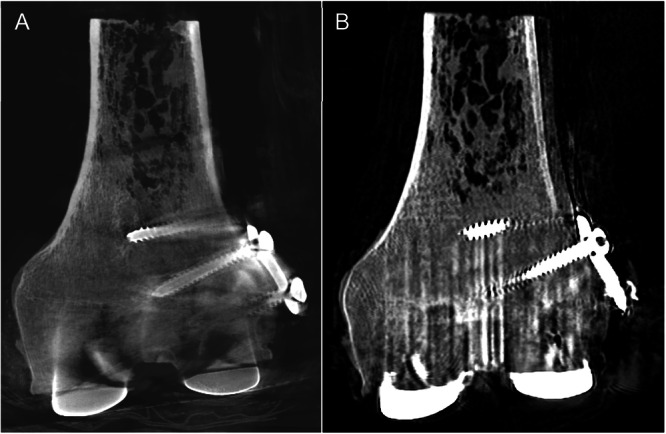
CT scans of a distal femur with periprosthetic fracture fixation plate and screws adjacent to a femoral component from total knee arthroplasty, acquired using a weight‐bearing CT scanner (A) and conventional CT scanner (B).
